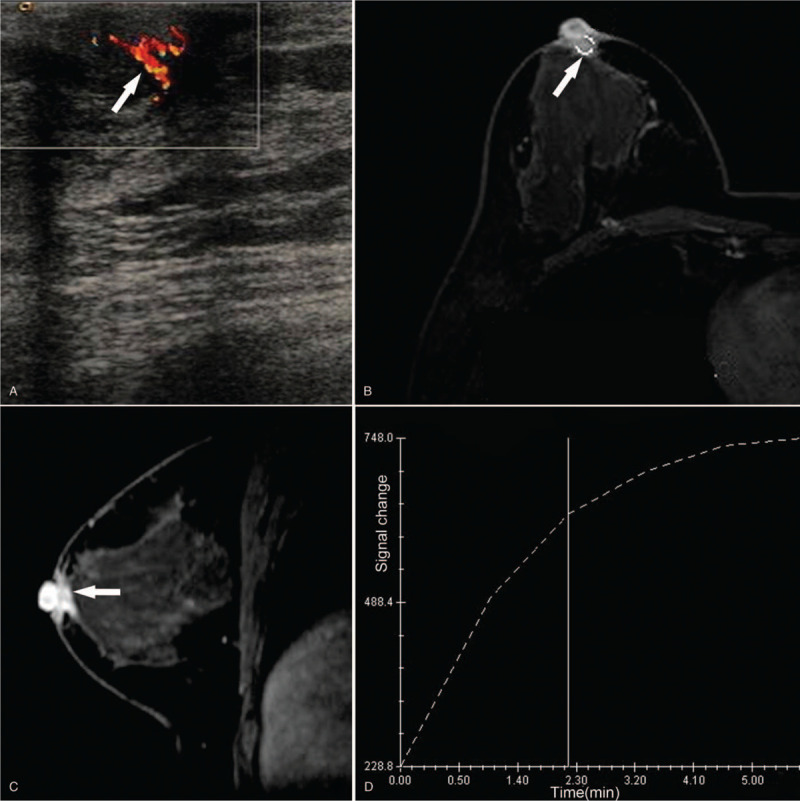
Case 2, right breast color Doppler ultrasound and MRI examination. Notes: (A). Image of Color Doppler ultrasound examination. White arrow points to the hypoechoic mass area behind the nipple. (B). Cross-section image of MRI. The white arrow indicates a dense mass behind the nipple. (C): Sagittal image of an MRI. The white arrow indicates a dense mass with long spicules behind the nipple. (D): The time-signal intensity curve of the mass region is of plateau type. MRI = magnetic resonance imaging.
